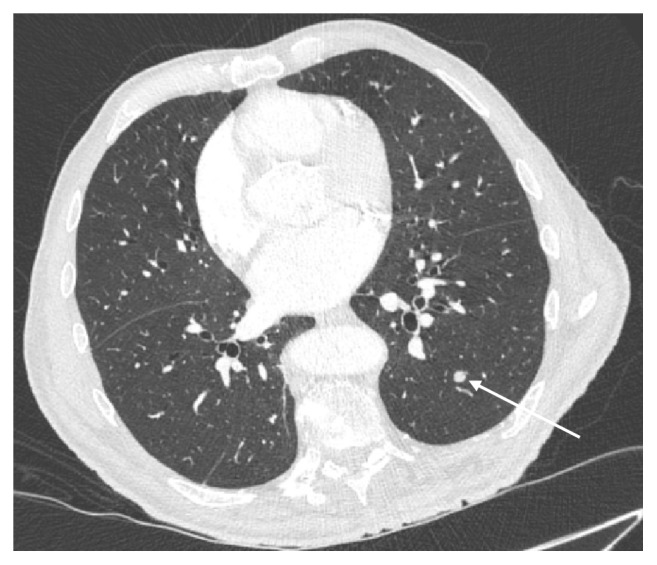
Axial CT image of the chest with lung windows revealing a pulmonary nodule in the posterior basal segment of the left lower lobe (white arrow).
